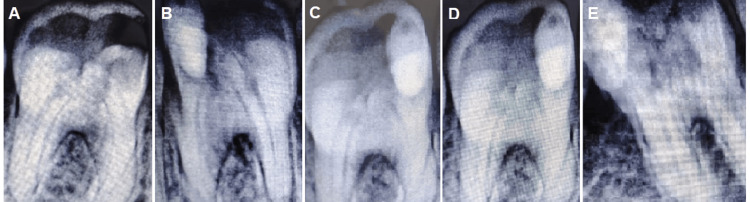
Radiographic evaluation of G4 at different time periods Original radiographic images taken during the study A. Preoperative showing proximal caries in molar tooth. B. Immediate post-operative radiograph after restoration. C. Image after one month showing initiation of reparative dentin formation. D. Image after three months showing increased reparative dentin formation. E. Image after six months showing a well-defined layer of reparative dentin under the restoration.
